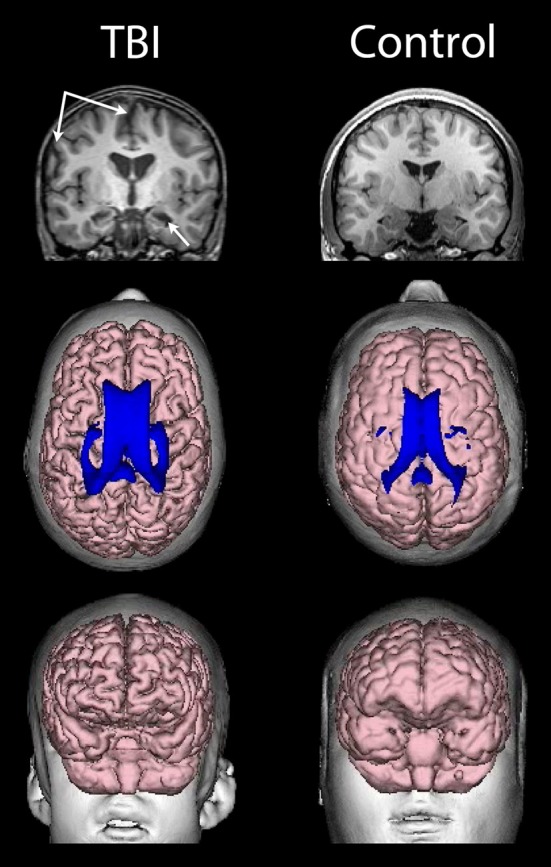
(Top) T1-weighted coronal images of a TBI patient on the left and an age-matched control on the right, both young adolescent males. Upper left-hand arrows point to a prominent interhemispheric fissure and cortical sulci, reflective of whole brain volume loss and generalized cerebral atrophy in the TBI patient. The lower arrow points to an atrophic hippocampus and dilated temporal horn, bilaterally in the TBI patient. (Middle) Dorsal view of a 3-D reconstruction of the ventricle in shown in blue superimposed on the flesh-tone brain surface 3-D reconstruction. Calculating whole brain volume and dividing it by total ventricular volume and multiplying by 100 results in a ventricle-to-brain ratio (VBR) of 5.55, which is markedly deviant from normal, which in typical developing controls is generally in the range of 1.5 with a 0.5 standard deviation [see Blatter et al. (1995) and Chang et al. (2005)]. The control subject VBR was 1.45. Frontal view of the 3-D reconstructed brain (Bottom) of the individual with TBI showing global frontal atrophy with visibly larger cortical sulci compared to the age-match control subject on the right, again reflective of generalized cerebral atrophy. Increased VBR reflects this type of global brain volume loss, ventricular enlargement, gyral shrinkage and sulcal enlargement.
